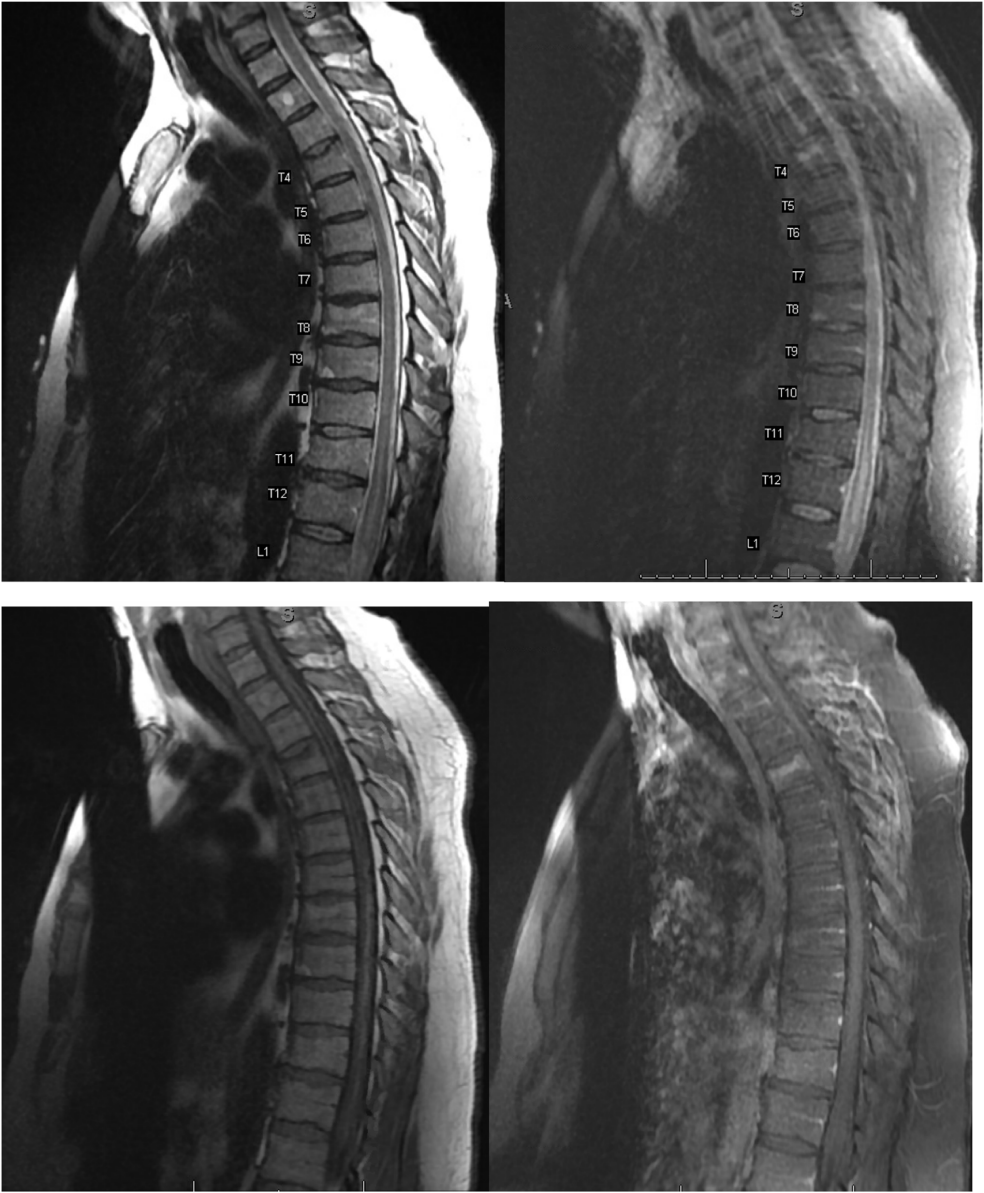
MRI Thoracic spine demonstrating abnormally increased T2 hyperintensity with expansion as seen on Sagittal T2 (top left) and Sagittal STIR (top right) extending from approximately the T10 vertebral level to the conus medullaris which terminates at approximately L1. Given lack of enhancement as demonstrated by the Sagittal T1 (bottom left) and Sagittal T1 post contrast (bottom right), this is most consistent with cord edema. No convincing evidence of cord signal abnormality above these levels. Considering the normal brain MRI, this abnormality most likely represents acute transverse myelitis.
